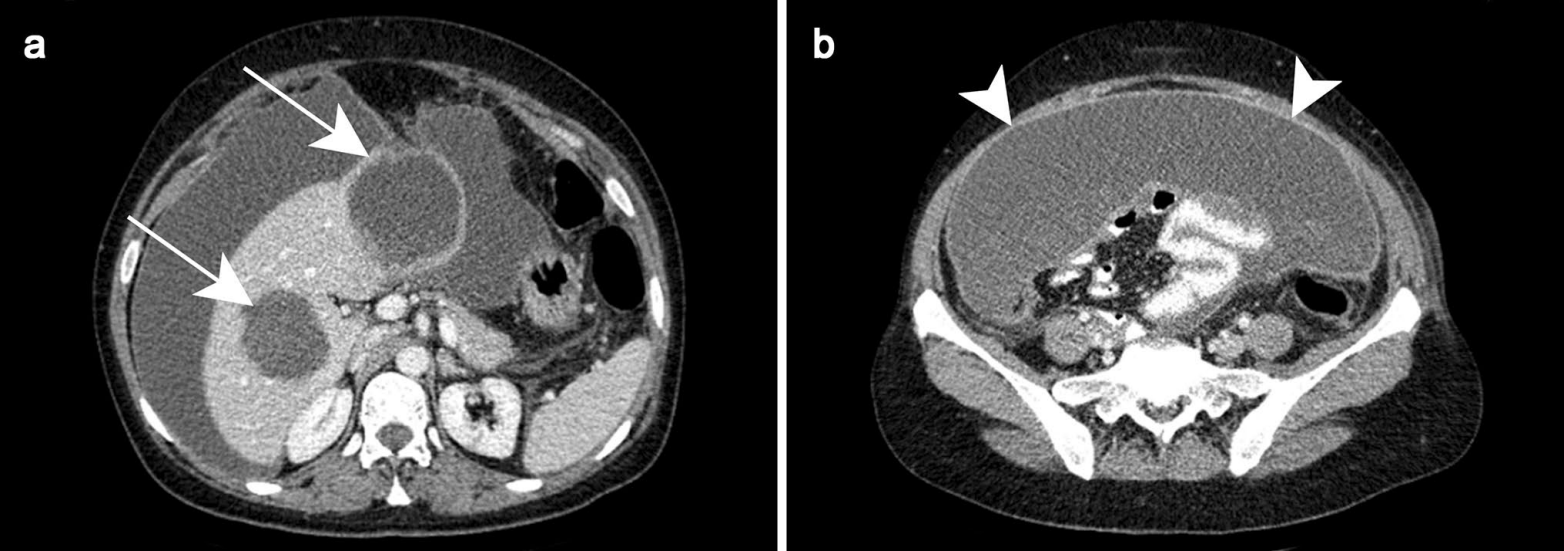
Peritonitis due to a ruptured hydatid cyst in a 45-year-old female patient with acute upper abdominal pain. Axial contrast-enhanced CT of the upper abdomen (a) demonstrates two hepatic cysts with irregular borders in the right and left liver lobe (white arrows); the latter reaches the anterior liver surface. Axial contrast-enhanced CT of lower abdomen (b) shows a significant amount of intraperitoneal fluid with a thickened enhancing peritoneum (white arrowheads)
